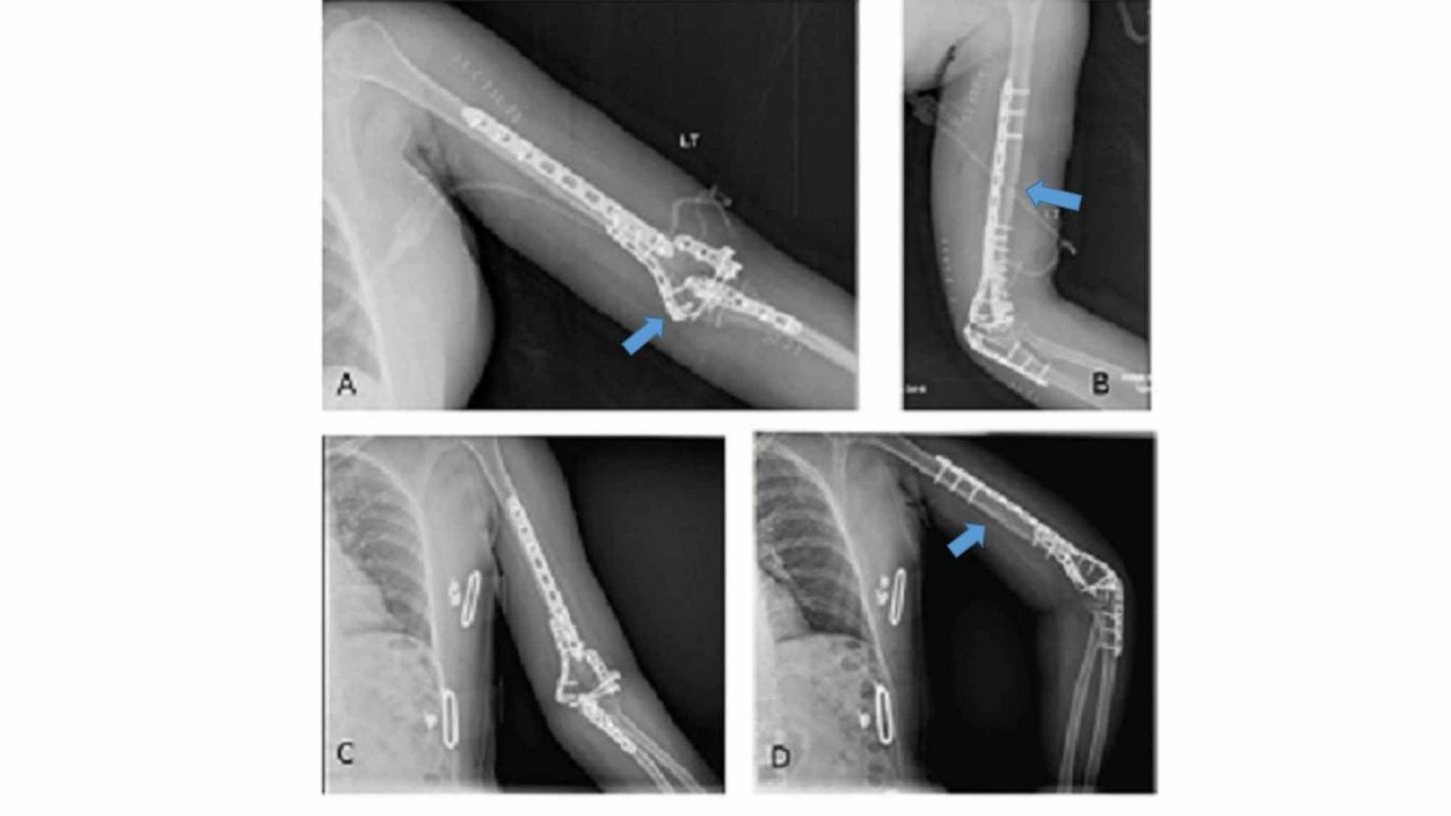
Anteroposterior (3A) and lateral (3B) radiograph showing fixation in the postoperative period and the healed fracture of shaft of the humerus (3C, 3D) at 12 months following surgery.
